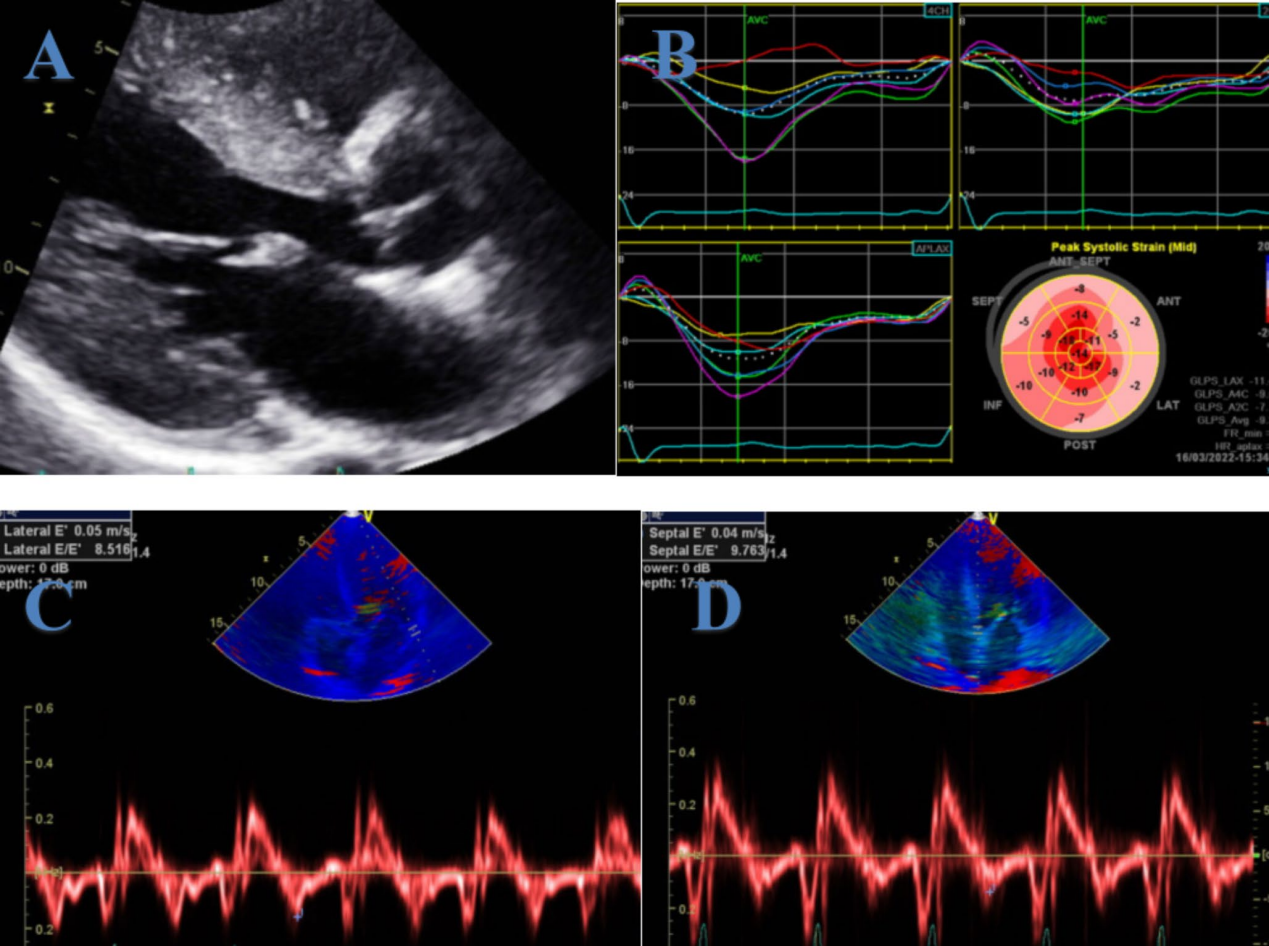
Echocardiography of a patient with cardiac amyloidosis. A: Parasternal long-axis view showing a thickened left ventricular wall. B: Global longitudinal strain of apical sparing pattern. C and D: Tissue doppler consistent with decreased diastolic function
